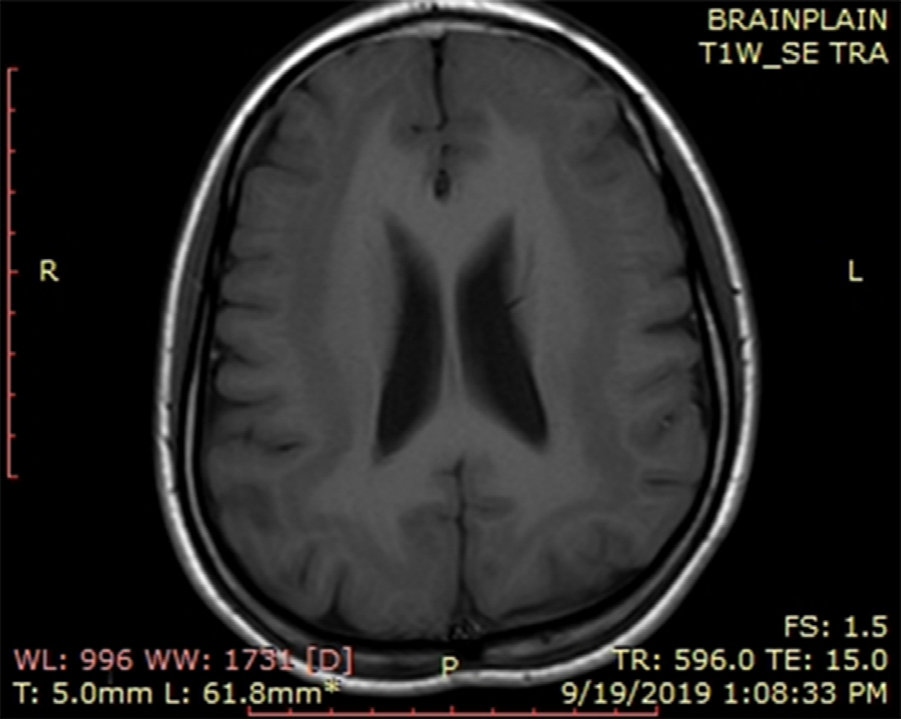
MRI brain plain T1WI showing continuous subcortical band of heterotopic grey matter.
